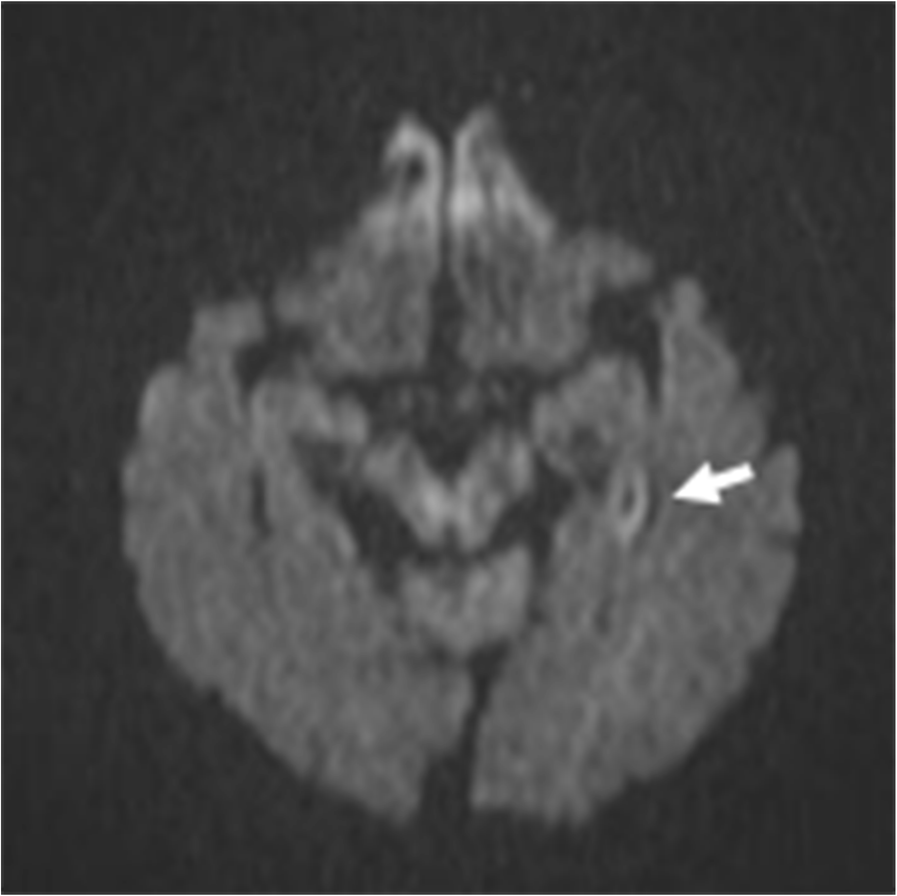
(Axial) Diffusion-weighted images (DWIs). DWI magnetic resonance imaging scans showing a high-intensity lesion in the dorsal part of the left hippocampal body (arrow).
